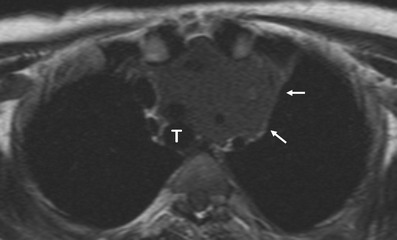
Axial T1-weighted MR image of a 16-year-old man with a solid, large mass (*arrows*) in the anterior and superior mediastinum. Supra-aortic trunks are almost completely surrounded by the lesion and trachea (*T*) is displaced to the contralateral side by the lesion. Pathological analysis demonstrated a T-cell lymphoblastic lymphoma
